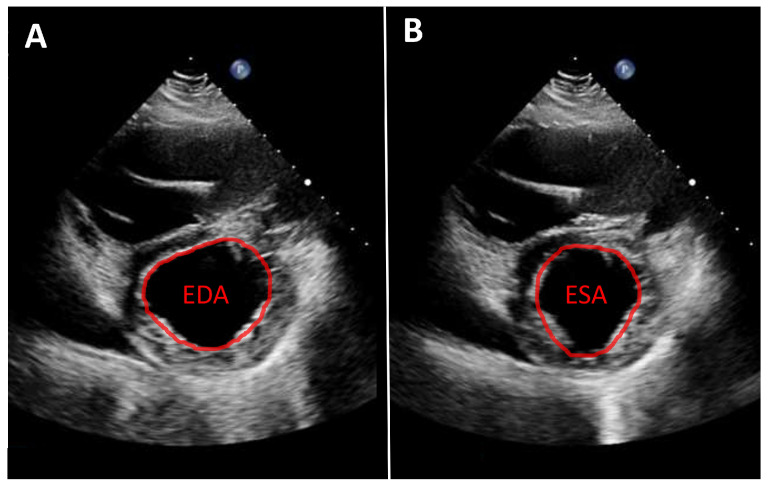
Parasternal short-axis view. Measurement of fractional area change (FAC) in a patient with an LVAD. (A): Planimetry of the end-diastolic area (EDA). (B): Planimetry of the end-systolic area (ESA). Calculation of FAC: FAC = (EDA − ESA)/EDA.
